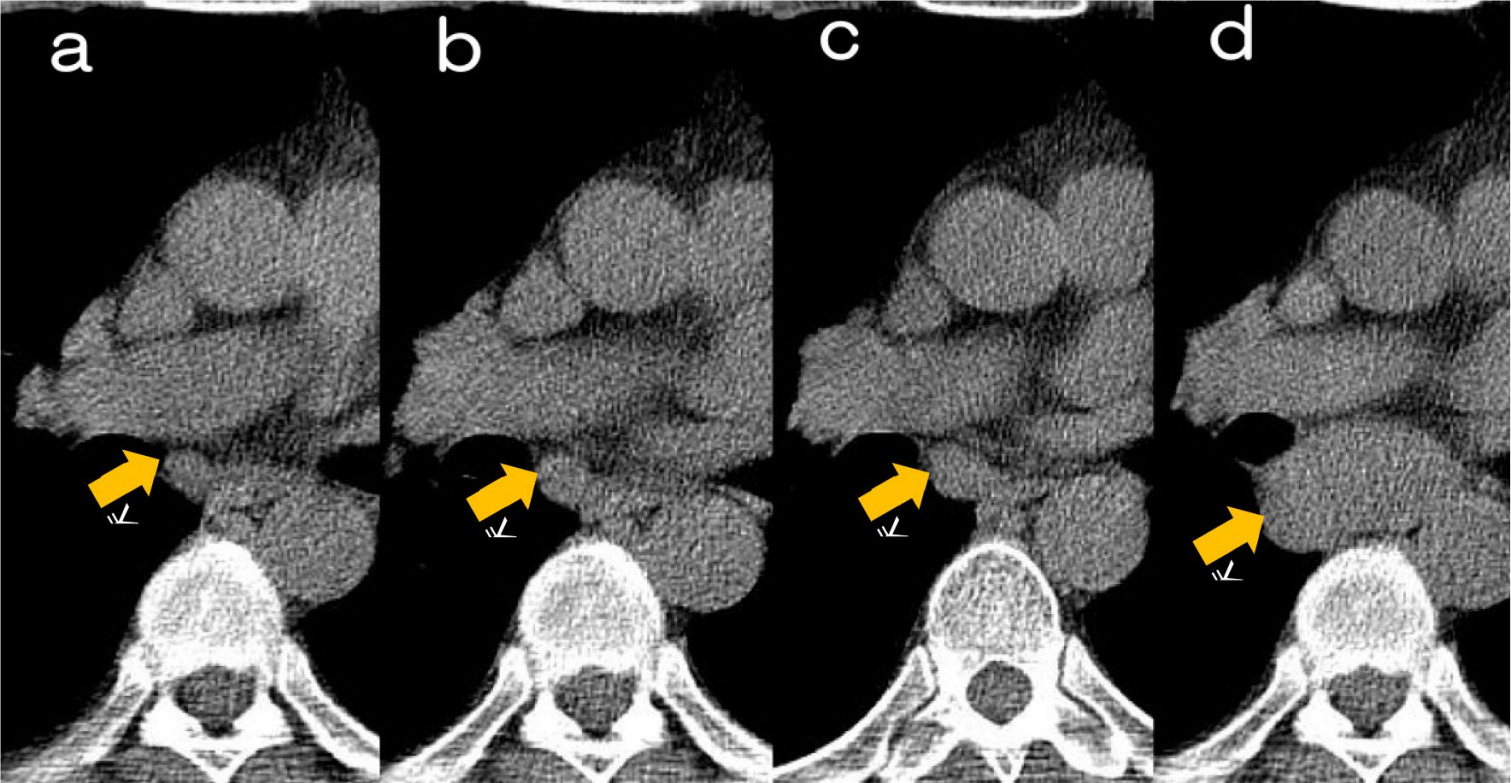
Case with temporal enlargement of mediastinal lymph nodes. a–d Series of low-dose CT screening images 3 years prior, 2 years prior, 1 year prior, and at the time of diagnosis reveal the progression of findings. a A round soft tissue nodule is noted adjacent to the intermediate bronchus (mediastinal lymph node #7) 3 years prior the time of diagnosis. b, c The round soft tissue nodule showed mild enlargement 2 years prior to the diagnosis (b), followed by further enlargement 1 year prior (c). d At the time of diagnosis, CT shows a large mass with 7 cm in diameter, extending from the tracheal bifurcation to the surrounding vicinity of the opposite main bronchus. Ultrasound-guided bronchoscopic needle biopsy reveals the diagnosis of “mediastinal type” primary lung adenocarcinoma, cT0N3M0. Low-dose CT images should be interpreted with caution, as the image quality of soft tissues such as the mediastinum may degrade, posing challenges for evaluation. Even with low-dose imaging, ensuring image quality sufficient for evaluating soft tissues is desirable
